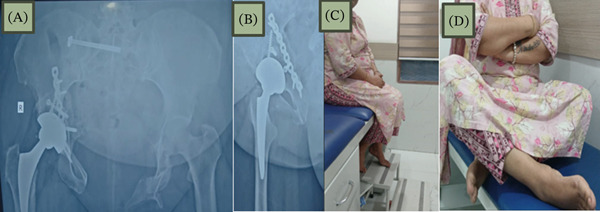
(A, B) Anteroposterior pelvic radiographs at 2‐year follow‐up demonstrating stable osseointegration of the acetabular and femoral components. (C, D) Clinical photographs obtained at 2 years postoperatively showing a good range of motion.
